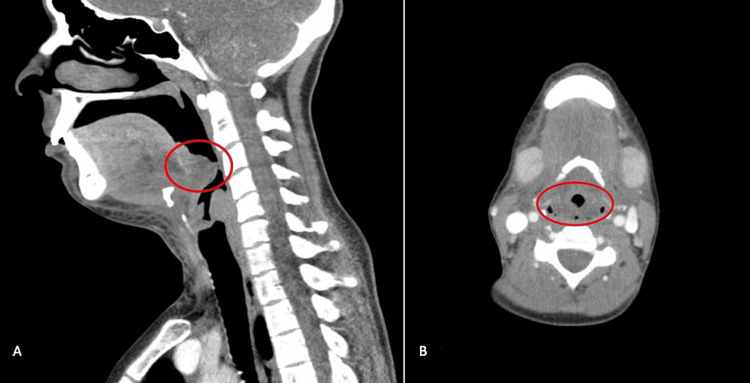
CT soft tissue neck with contrast. (A) Sagittal view. (B) Axial view. Diffuse enlargement edema involving the epiglottis, lingual tonsil, and arytenoids with moderate narrowing of the hypopharynx. CT: computed tomography.
